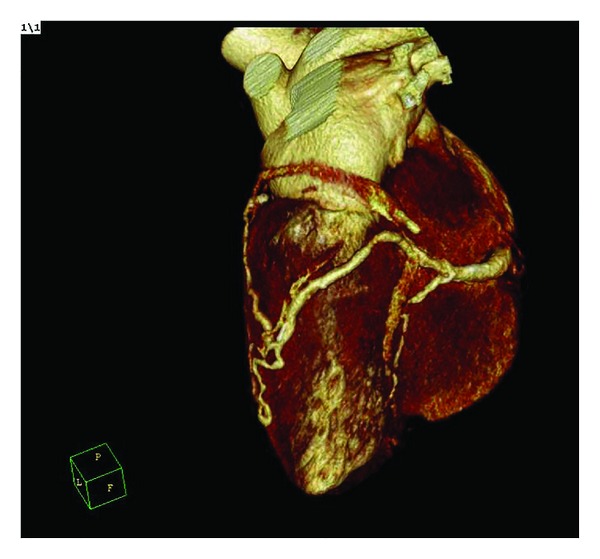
Multislice computed tomography. Volume rendering image shows that right coronary artery preceded in its regular pathway and gave branches to the left coronary system and then terminated with a fistula into the left ventricle.
